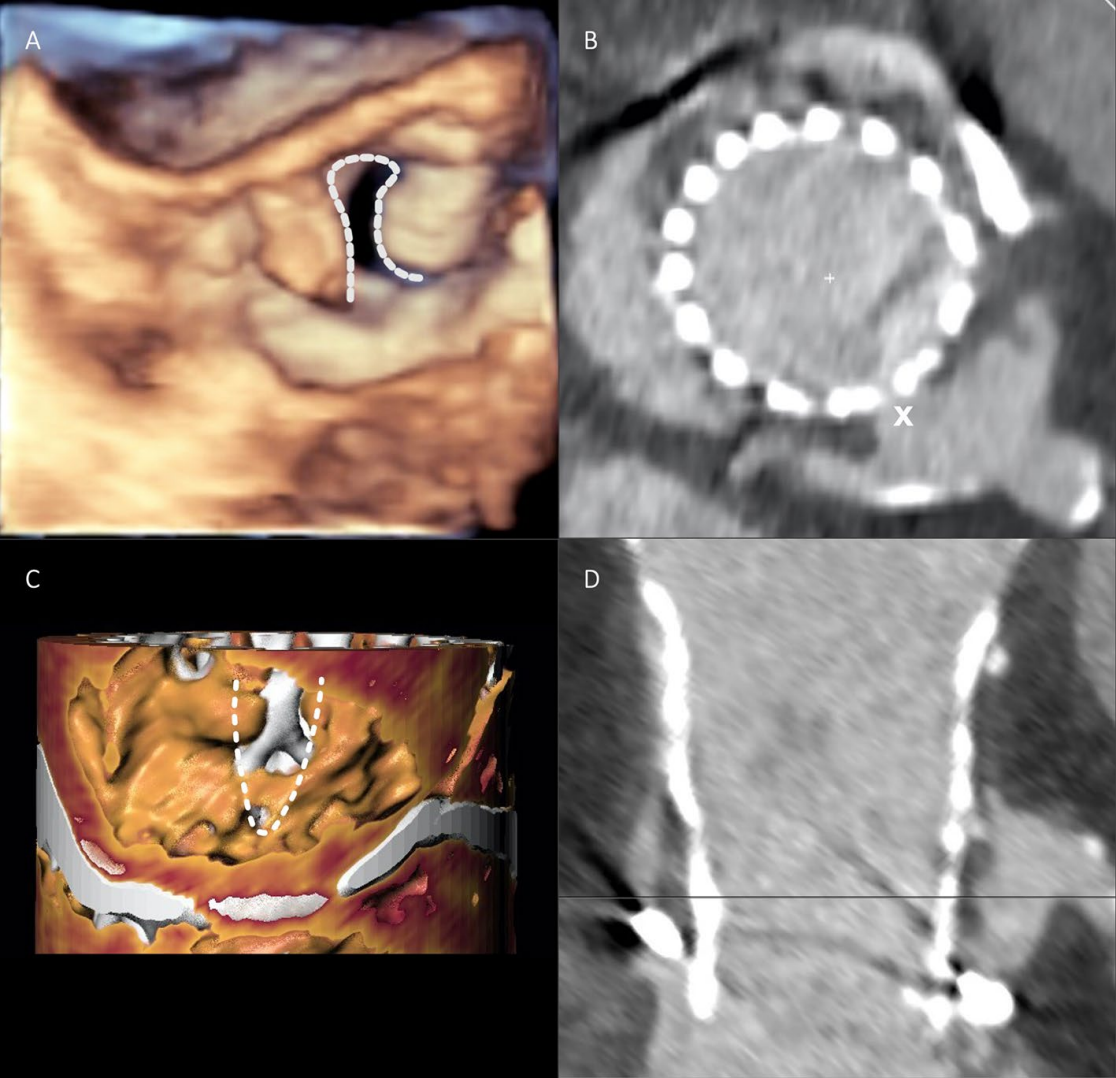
Successful BASILICA. A Confirmation of successful leaflet laceration by peri-procedural transesophageal echocardiography. B Lacerated leaflet (x) in a patient with Sequestered Sinus, post-TAVI CT. C Confirmation of lacerated leaflet (dashed line), post-TAVI three-dimensional CT reconstruction. D Post-TAVI CT in a patient with Sequestered Sinus (CoreValve Evolut R in Sorin Mitroflow) after BASILICA. *BASILICA* bioprosthetic or native aortic scallop intentional laceration to prevent iatrogenic coronary artery obstruction, *CT* computed tomography, *TAVI* transcatheter aortic valve implantation
